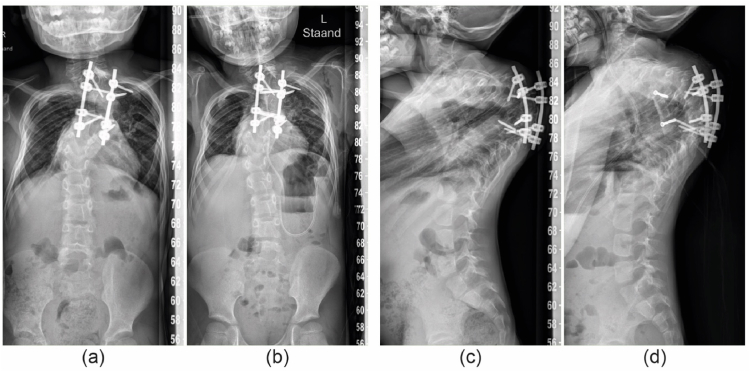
Patient 6; (a and b) Pre and post operative AP radiographs, (c and d) Pre and post operative lateral radiographs showing the 3D shaped allograft fixated with screws.
